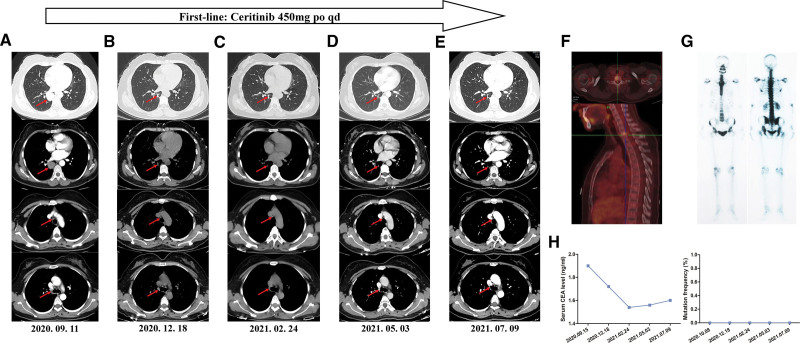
Dynamic monitoring of the response of the patient with lung adenocarcinoma to ceritinib. (A) Chest computed tomography (CT) scans on September 11, 2020 revealed the presence of a mass in the lower lobe of the right lung and enlargement of mediastinal and right hilar lymph nodes. Significant (December 18, 2020) (B) and consistent (February 24, May 3 and July 9, 2021) (C–E) reduction of the tumor volume and enlargement of mediastinal/right hilar lymph nodes were observed by the follow-up CT scans after the first-line therapy with ceritinib. (F) Positron emission tomography computed tomography (PET-CT) scanning on September 17, 2020 revealed bone metastasis at the C7 vertebral body. (G) Emission computed tomographic (ECT) bone scanning after treatment with ceritinib for 6 months showed bone metastasis disappeared. (H) Dynamic change of serum carcinoembryonic antigen (CEA) level and intergenic region ALK mutation frequency in peripheral blood ctDNA during the treatment course.
